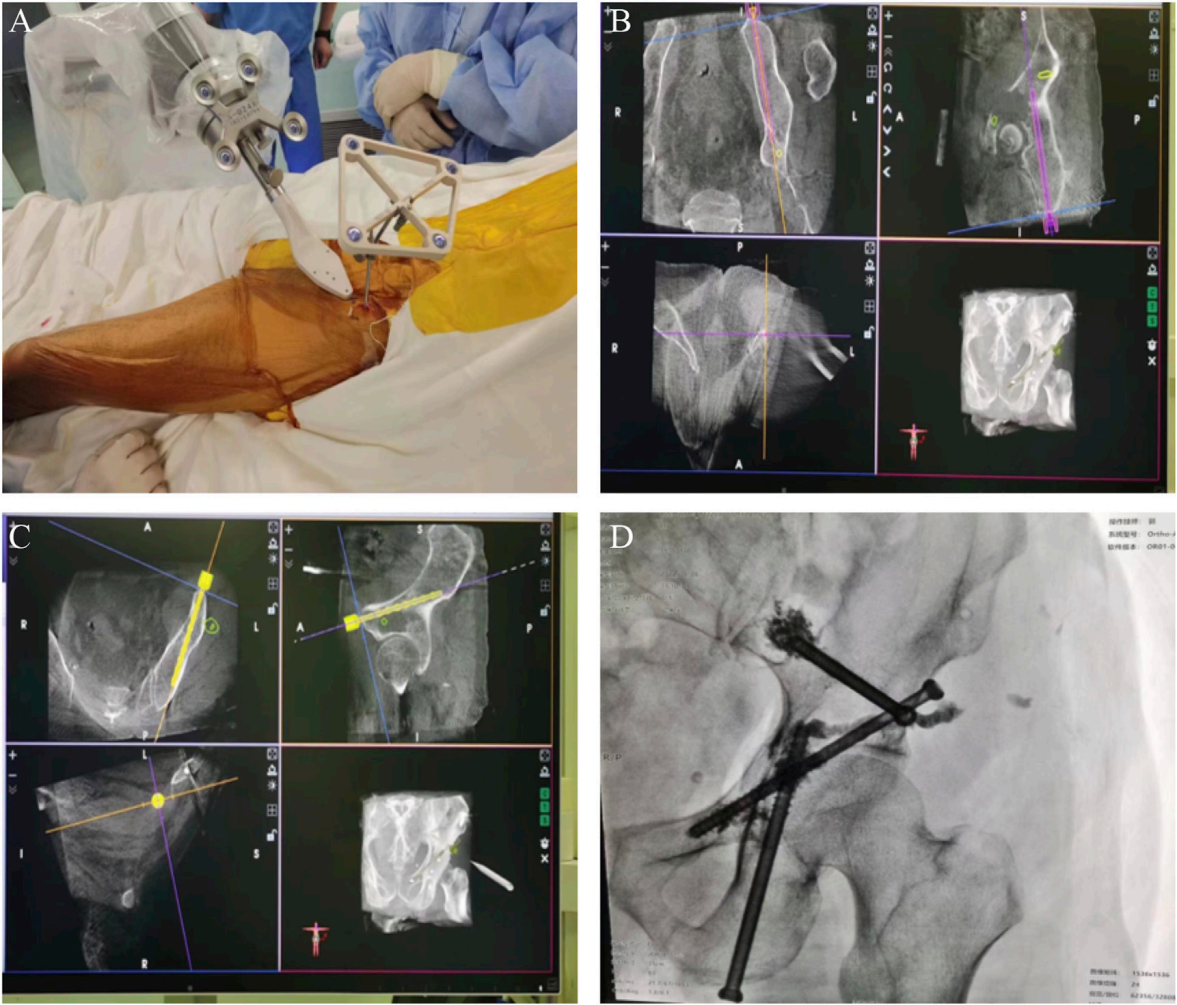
Intraoperative orthopedic robot for accurate positioning and imaging at the completion of surgery. (A–C) Accurately located screw channels with the help of orthopedic robot (D) X-ray radiographs of screw fixation and cement filling when the surgery was completed.
